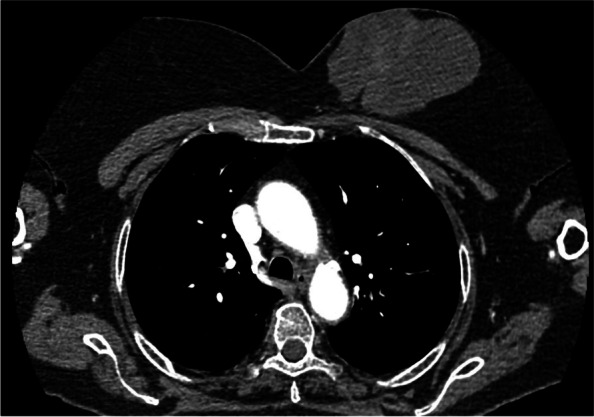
CT axial view of the chest showing huge breast cancer on the left side
